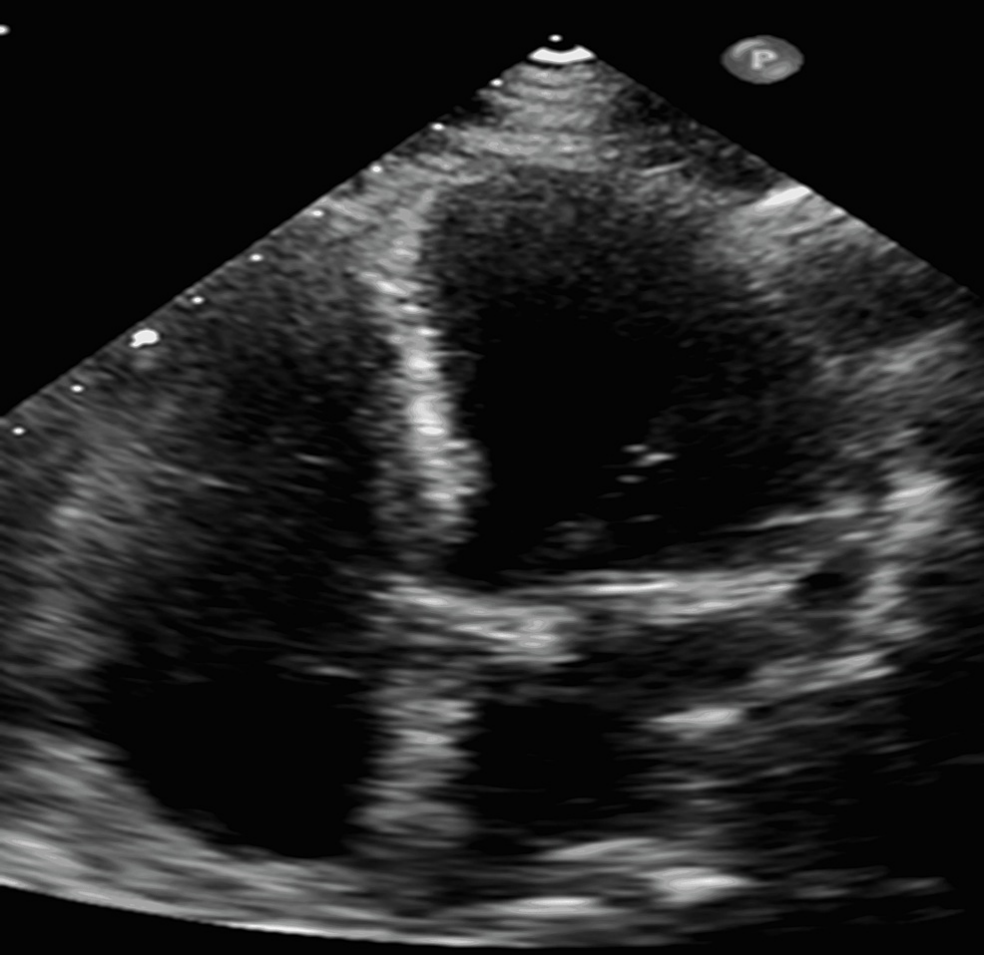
Echocardiogram findings after pericardiocentesis showing trivial pericardial effusion without signs of tamponade and improvement in LVEF. LVEF: Left ventricular ejection fraction.
